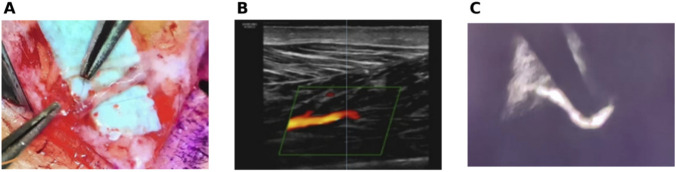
In vivo* implantation and imaging confirmation of graft patency. (A) Representative intraoperative view during graft implantation/anastomosis. (B) Color Doppler ultrasound demonstrating flow signal within the implanted graft/target vessel. (C) Representative angiographic image confirming graft/vessel patency.
